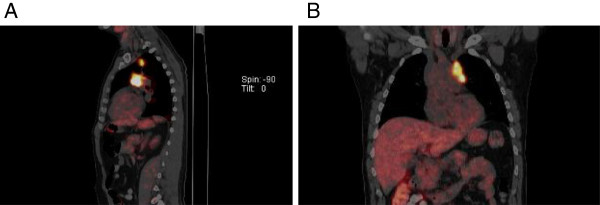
Whole-body FDG PET image in a fasting condition shows FDG accumulation in multiple prevascular, left hilar and subcarinal lymph nodes. There is no myocardial uptake of FDG. Sagital image of fasting FDG-PET (A). Coronal image of fasting FDG-PET (B).
